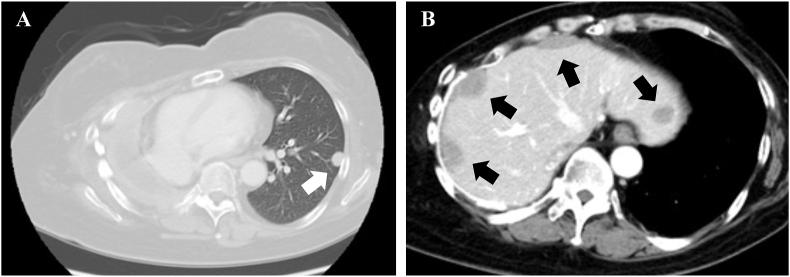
Chest computed tomography (CT) revealed multiple nodular lesions (A) in the left lung (white arrows) and (B) around the liver (black arrows) 8 years after complete resection of the thymoma with the right lung.
